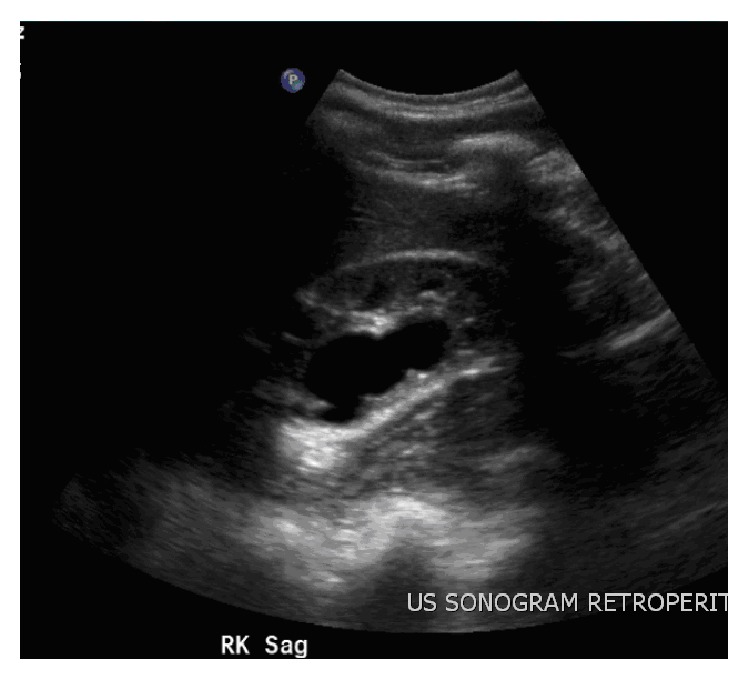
Ultrasound of right kidney read as pelviectasis and possible renal cysts.
